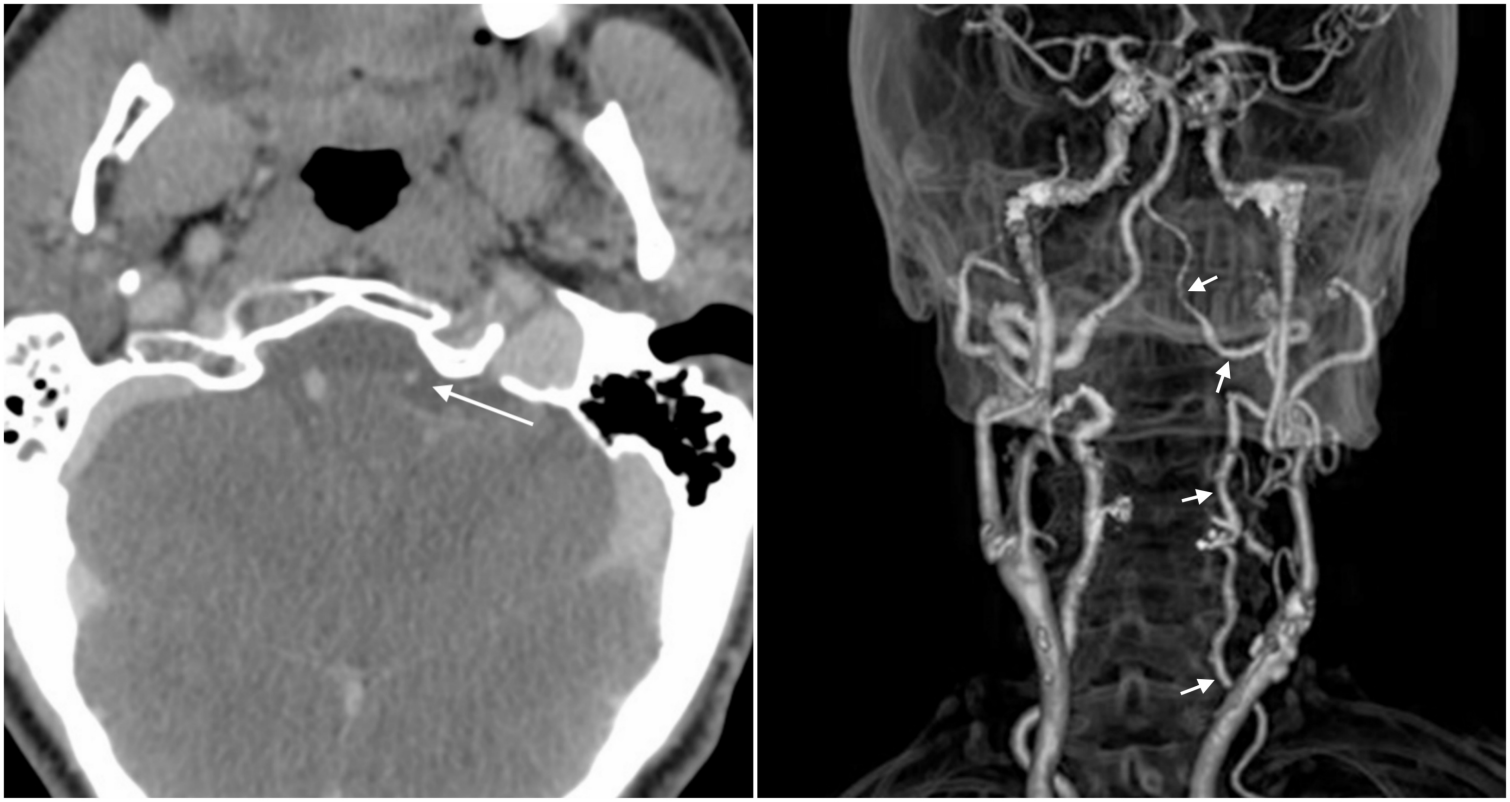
Computed tomography (CT) angiogram Left: Contrast CT scan showing the small diameter observed in the left vertebral artery (arrow). Right: CT 3D reconstruction displaying a hypoplastic left vertebral artery. This finding is observed from the origin of this artery (arrows).
